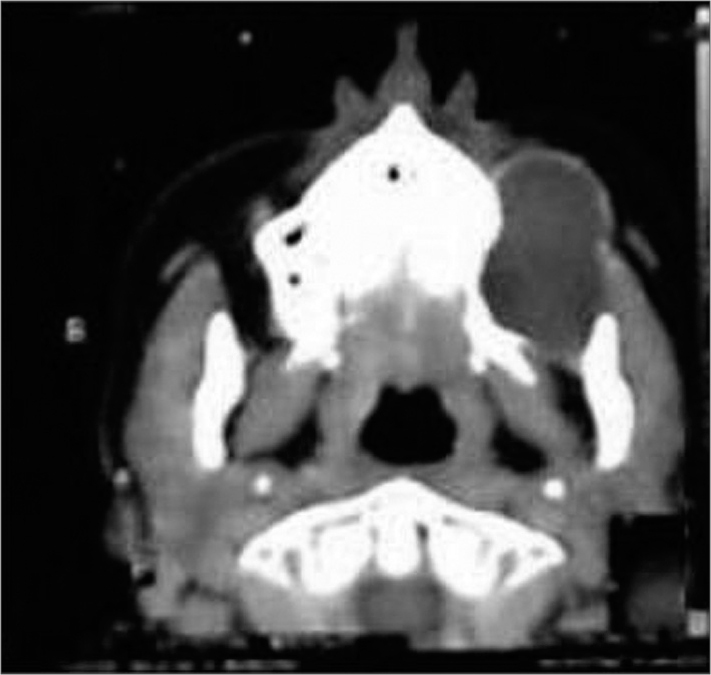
Computed tomography showing an intraosseous cyst in the left maxilla.
